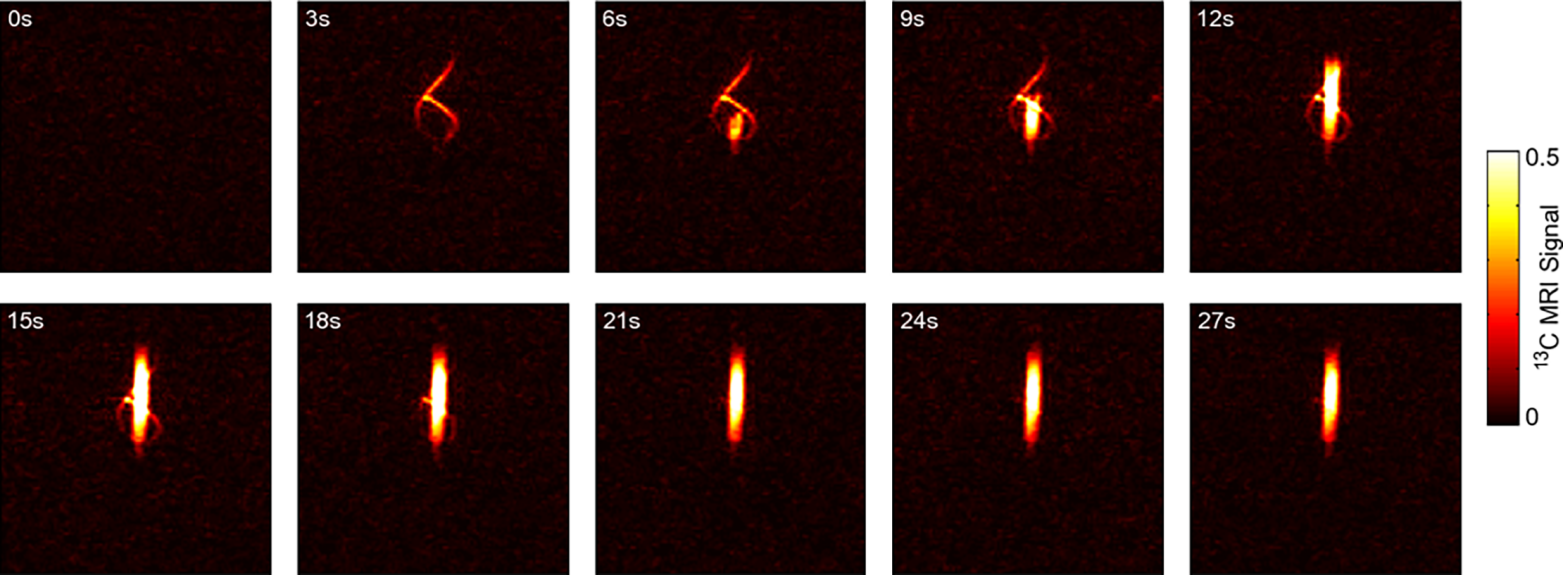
Dynamic ^13^C-MRI acquired during the injection of a SAMBADENA-produced agent. Ten images were recorded using Setup 2 with a repetition time of *T*_R_ = 3 s and an acquisition time of each image of ~500ms during the injection of ~ 600 μl tracer solution into a test object (HP solution: 5 mM HEA, 2 mM catalyst in H_2_O, *t*_h_ = 5 s; test object described in S4 Fig). Injection was started after the first scan and ended after the sixth (15s). Note that the injection was paused for MRI. After each scan, the magnetization was flipped back towards the longitudinal direction to conserve magnetization. The signal of all images was normalized with respect to the highest signal of image No. 6; the colour scale was trimmed to 0–0.5 of the maximum intensity to visualize the signal in the small hose. ^13^C-MRI parameters: 90/180°, RARE-factor: 38, FOV: (8.4cm)^2^, acquisition matrix of 96x96 px, interpolated to 256x256 px, in-plane resolution: 0.33 x 0.33 mm, one slice with a thickness of 6 cm, *T*_R_ = 0.487 s, *T*_E_ = 79 ms, acquisition time: 0.487 s.
